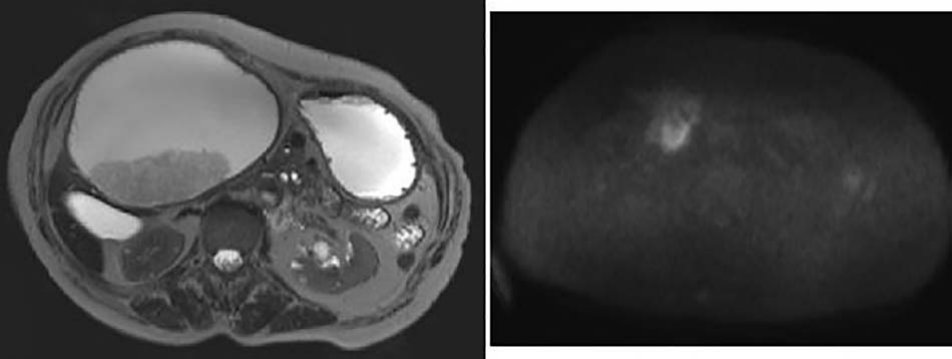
Giant cyst showed high signal intensity on T2-weighted images and fuller component in dorsal side showed high signal on diffusion-weighted images.
